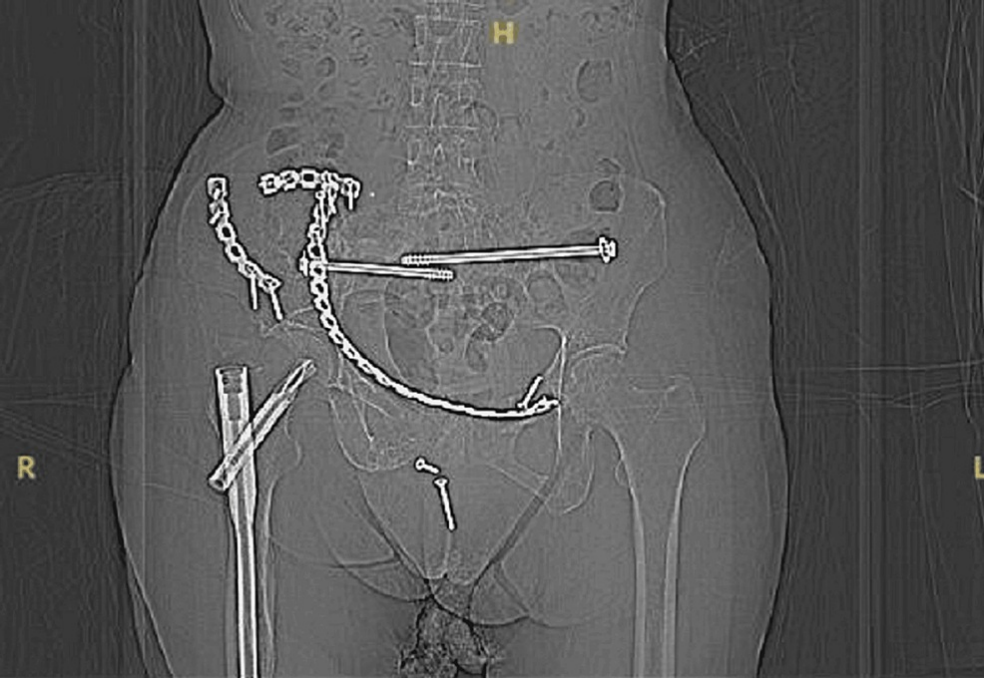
Radiograph upon admission showing the anterior pelvic bridge plate extending from the right ilium to the left pubic region, two reconstruction plates over the right iliac fossa, and three sacral screws inserted to fix bilateral sacral fractures. Three migrated screws were detected in the pelvic region.
